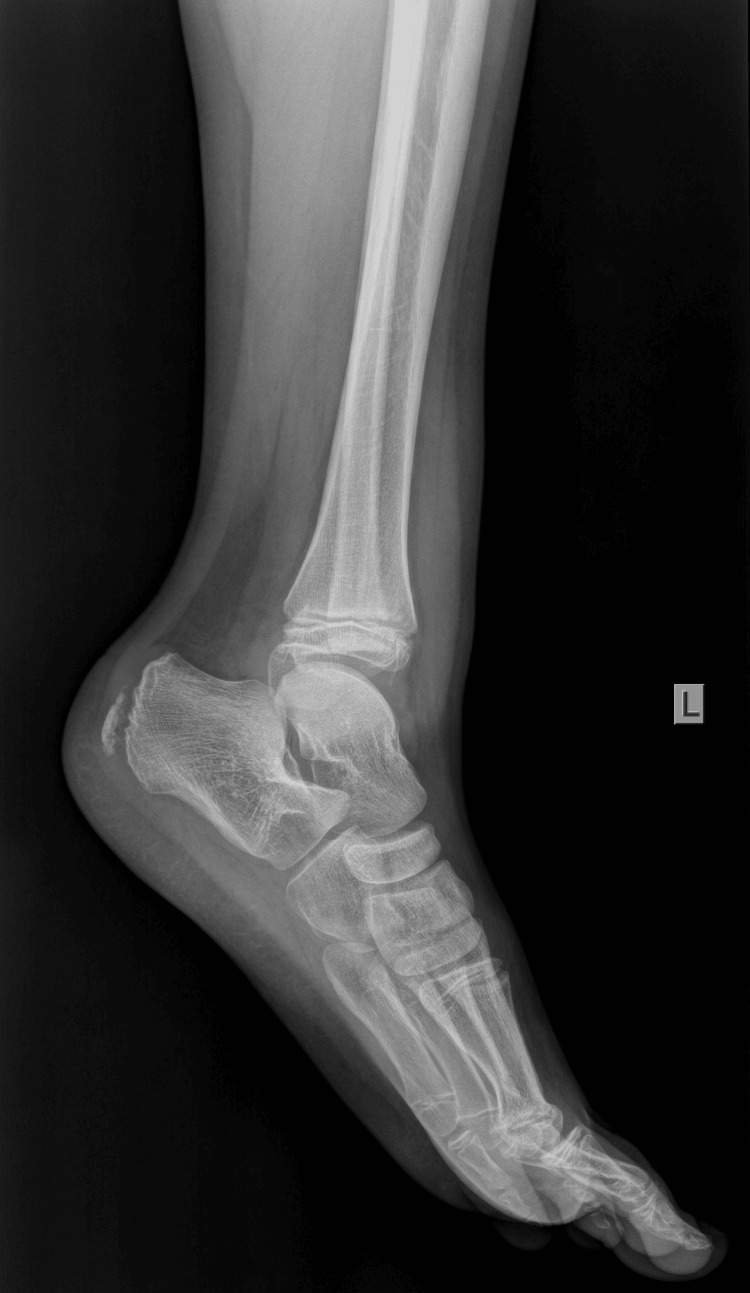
Initial X-ray lateral diagnosed as an ankle sprain, with soft tissue oedema of the lateral malleolus.
